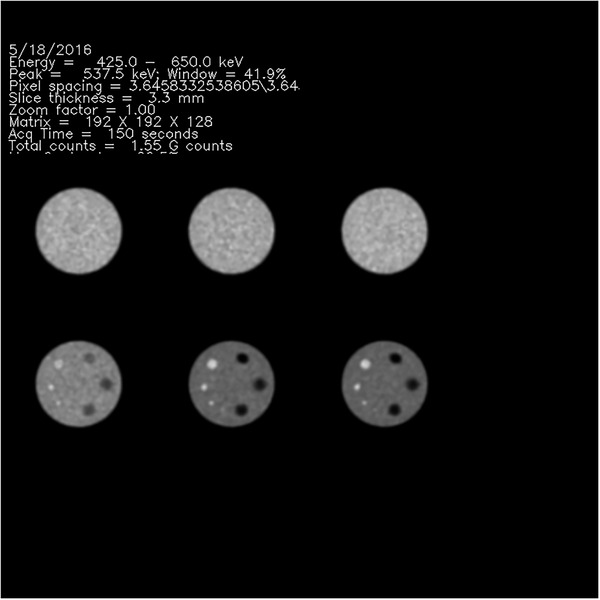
One of the jpg files of the automatically generated QA report for a standardized PET/CT data acquisition, which was used for the visual scoring of confidence of “hot” cylinders visibility
